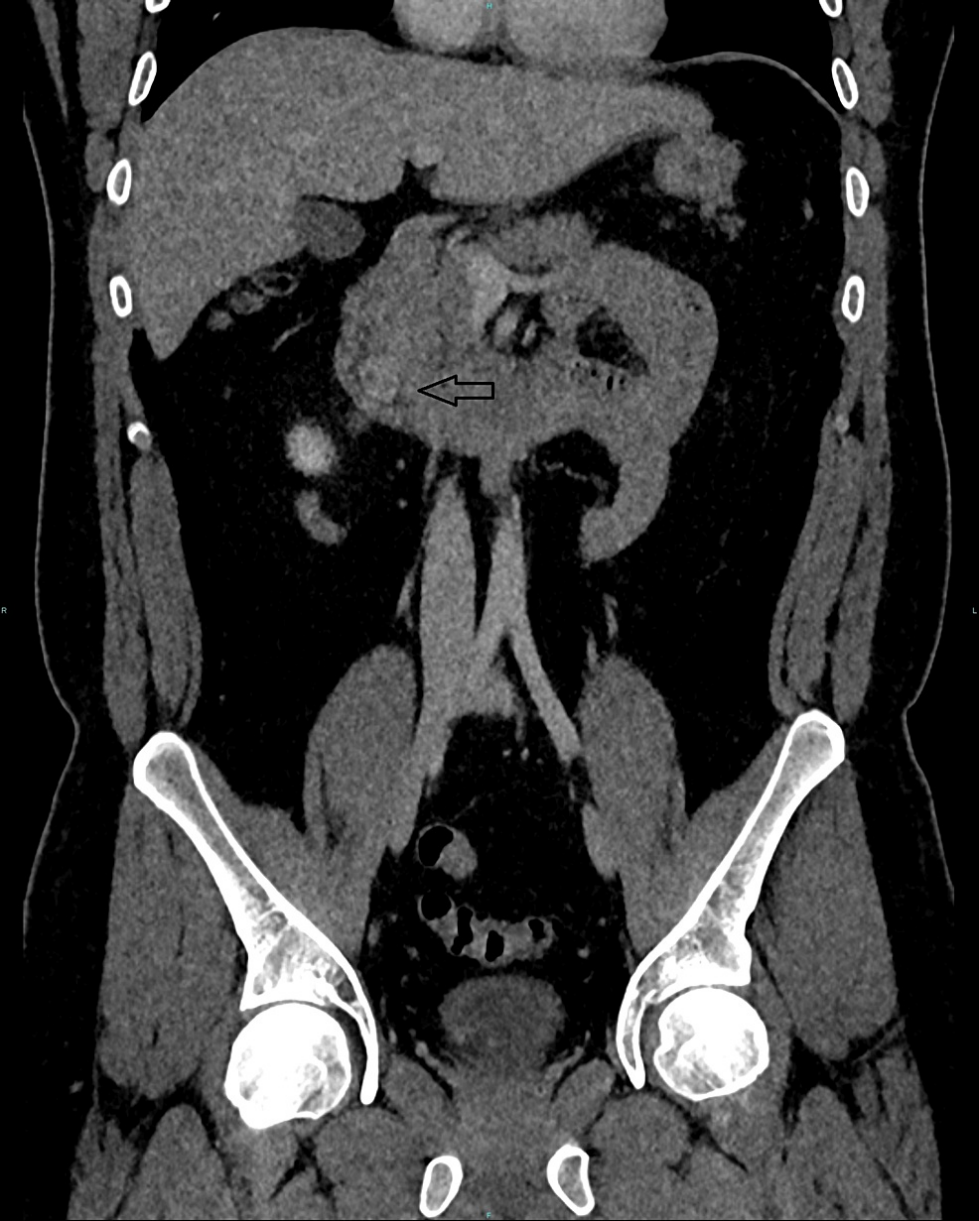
Duodenal gastrointestinal stromal tumour (GIST) on computed tomography (CT) angiogram of the abdomen, axial view Arrowhead points to the gastrointestinal stromal tumour (GIST)
